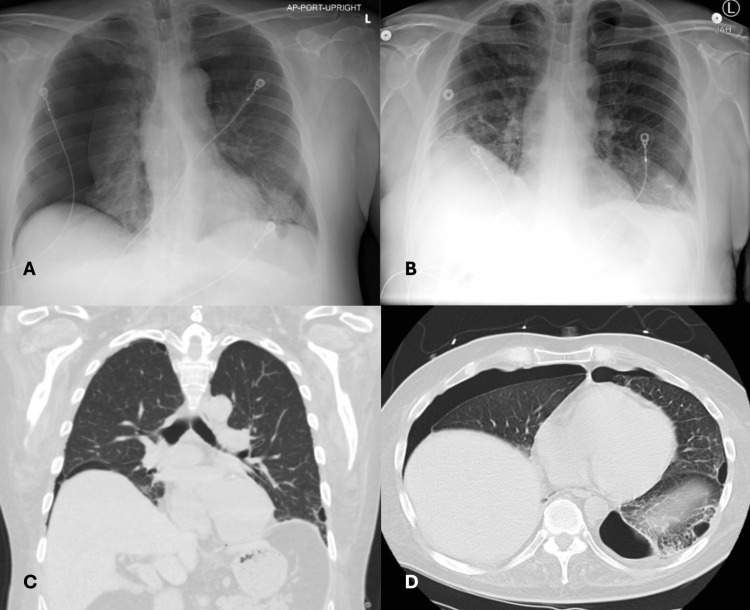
Initial and Subsequent Radiological Findings A) Chest radiography from initial presentation with bilateral pneumothoraces. B) Chest radiography from subsequent presentation with left apical pneumothorax. C) Computed tomography of the chest with lung cysts primarily at the bases. A few cysts are located at the apex. D) Sagittal plane view with bilateral pneumothoraces and lung bullae present at the left lung base.
